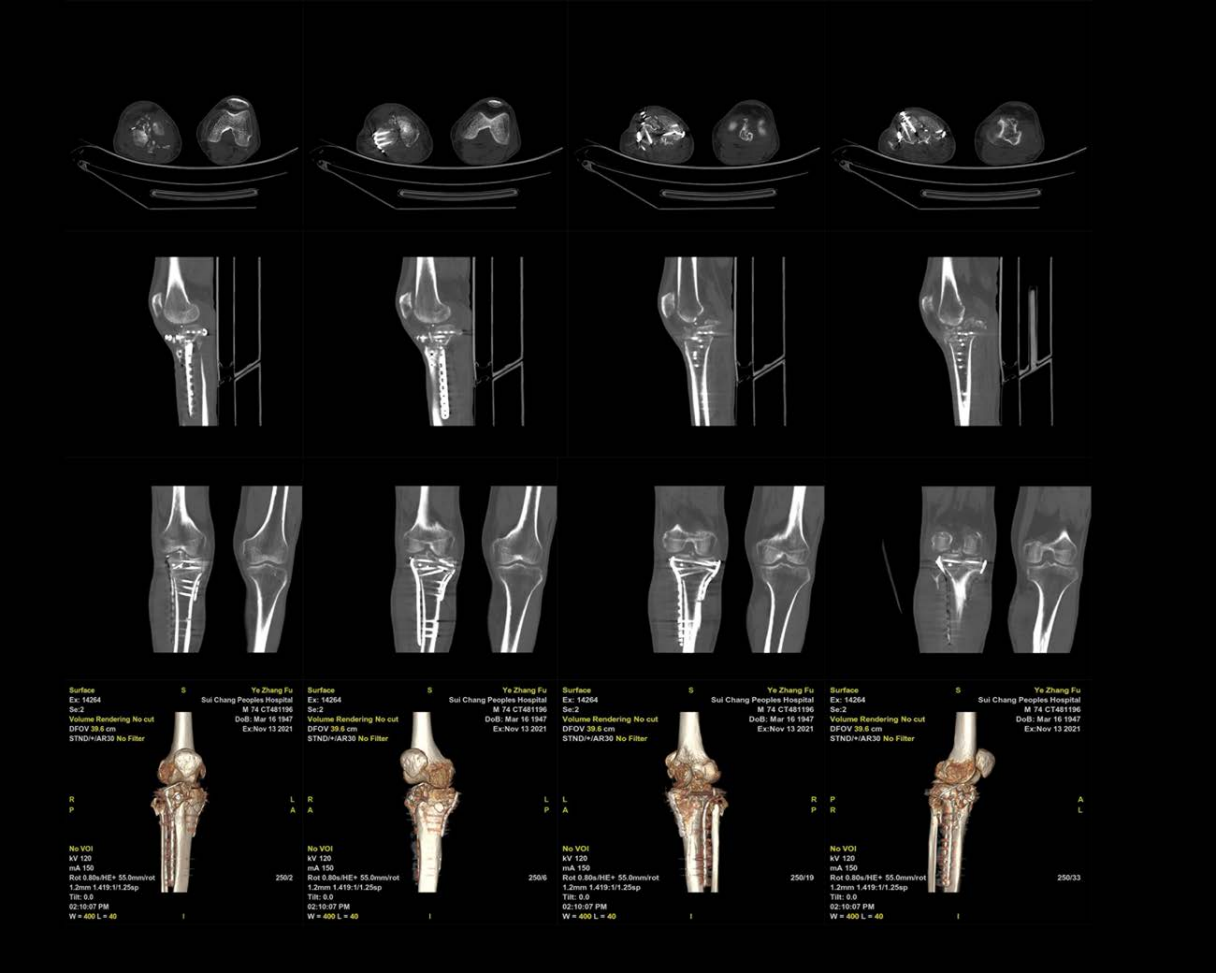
Computed tomography showed a small bony callus in the fracture area approximately 3 months after surgery (Fig. 5; date of examination: November 13, 2021). Axial (A; B; C; D), agittal (E; F; G; H), coronal (I; J; K; L), and 3-dimensional (M; N; O; P) CT.
